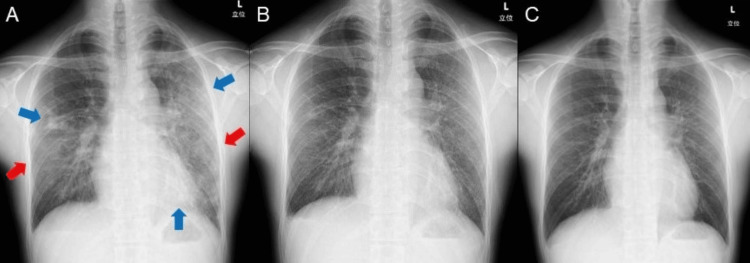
Time-course change in chest radiograph findings. (A) Chest radiograph obtained on the day of admission showing extensive ground-glass opacities (red arrows) and infiltrative shadows (blue arrows) in both lung fields; (B) Chest radiograph obtained on the 3^rd^ day of admission showing improvement in the pneumonic shadows; (C) Chest radiograph obtained on the 7^th^ day of admission showing further improvement in the pneumonic shadows.
